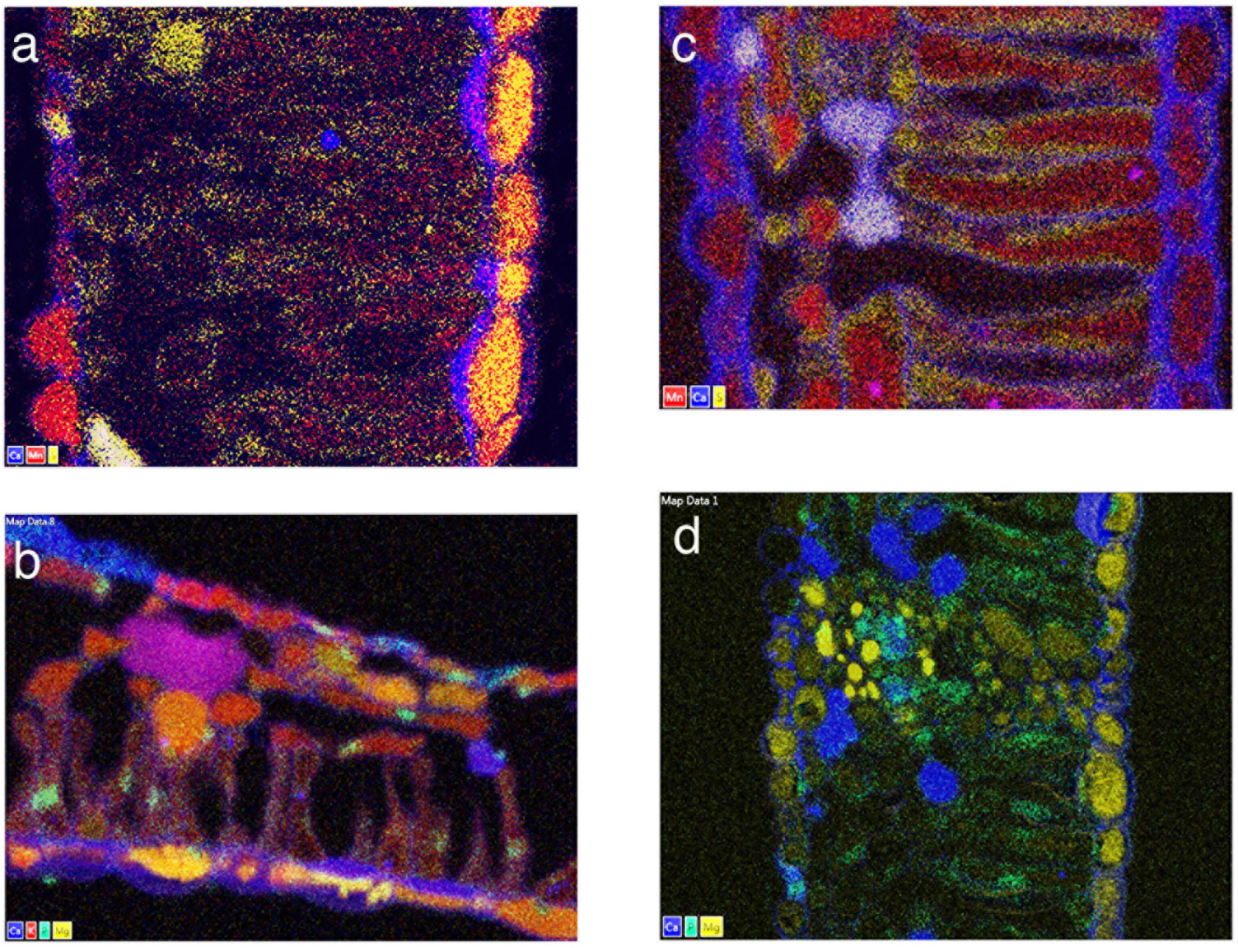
X-ray mapping data from Figs 2–4 combined here to highlight differences in multi-elemental co-deposition patterns in: a) SM/U, b) SM/D, c) RM/U, d) RM/D.
